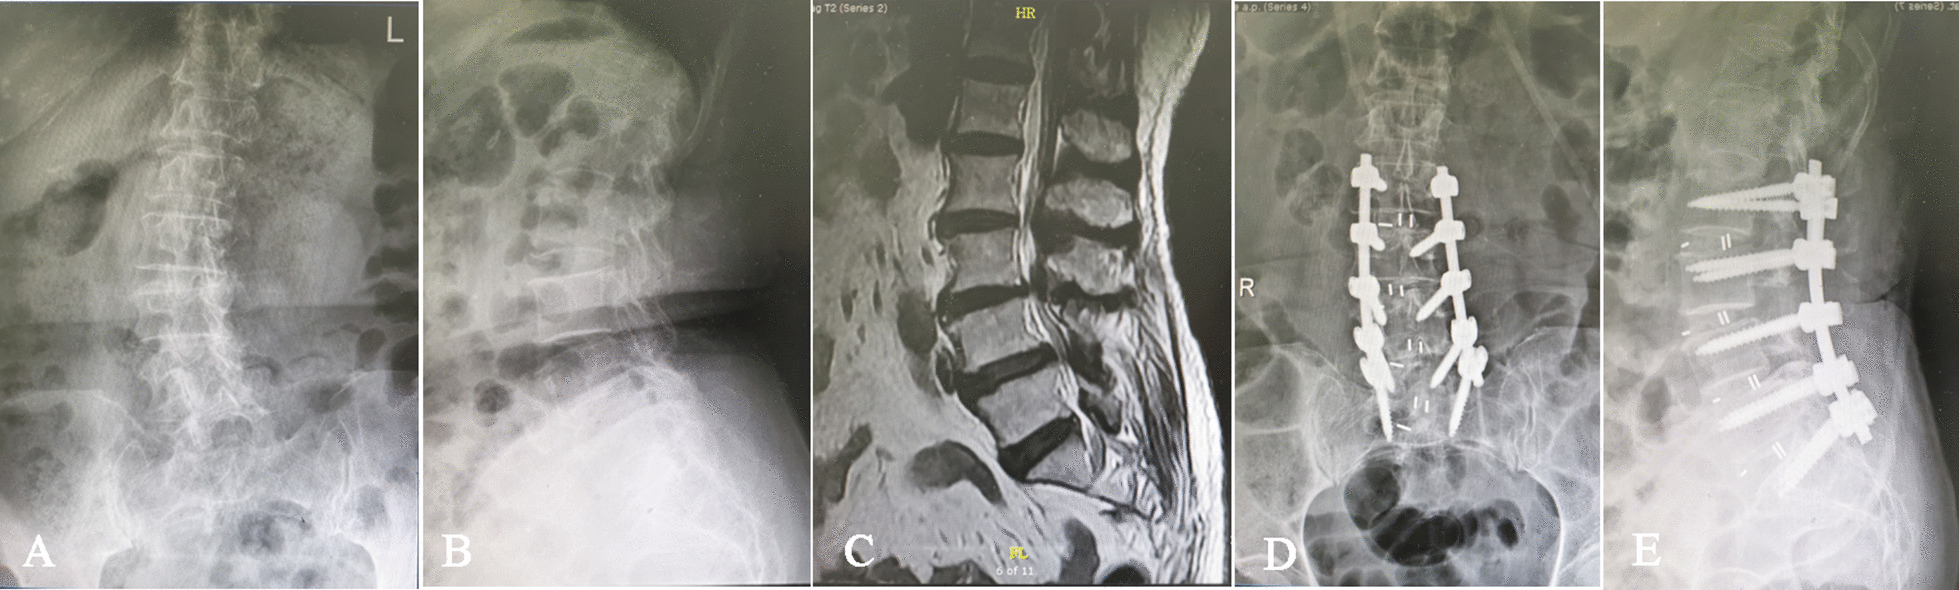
Preoperative lateral radiographic and MRI view (A–E) of a 75-year-old female with low back pain and intermittent claudication of both lower limbs for more than half a year. According to the preoperative standing long cassette coronal and sagittal radiographs, the Cobb angles and lumbar lordosis were 15° and 51°, respectively (A, B). The postoperative standing long cassette coronal and sagittal radiographs at final follow-up showed that the Cobb angle and lumbar lordosis improved from 15° to 6° and from 51° to 54°, respectively (D, E)
